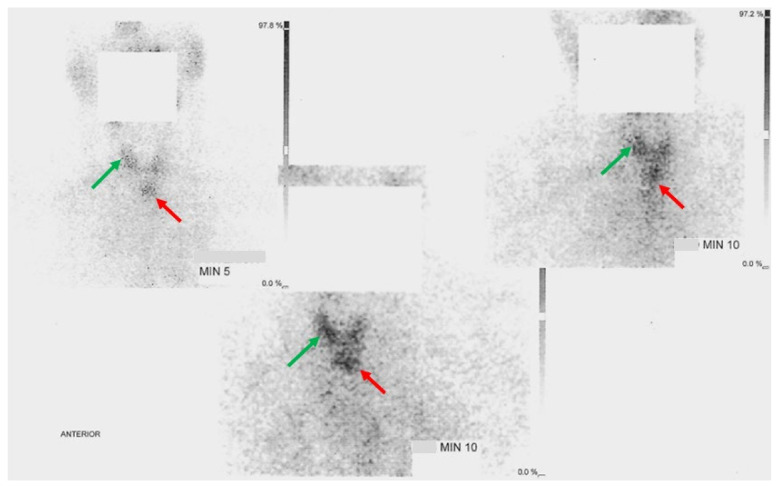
99m-Tc pertechnetate (148 MBq; effective dose 1.924 mSv) thyroid scintigraphy: an area of increased uptake in the upper part of the right thyroid lobe (orthotropic multinodular gland = green arrows) and an area of hyper-functional retrosternal thyroid tissue with hot nodules (ETTM = red arrows).
